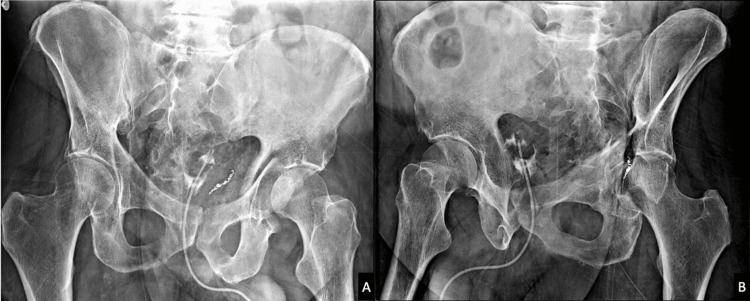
A) Iliac oblique B) obturator oblique radiographs of a 63-year-old male who sustained a column acetabulum fracture after falling from a height
